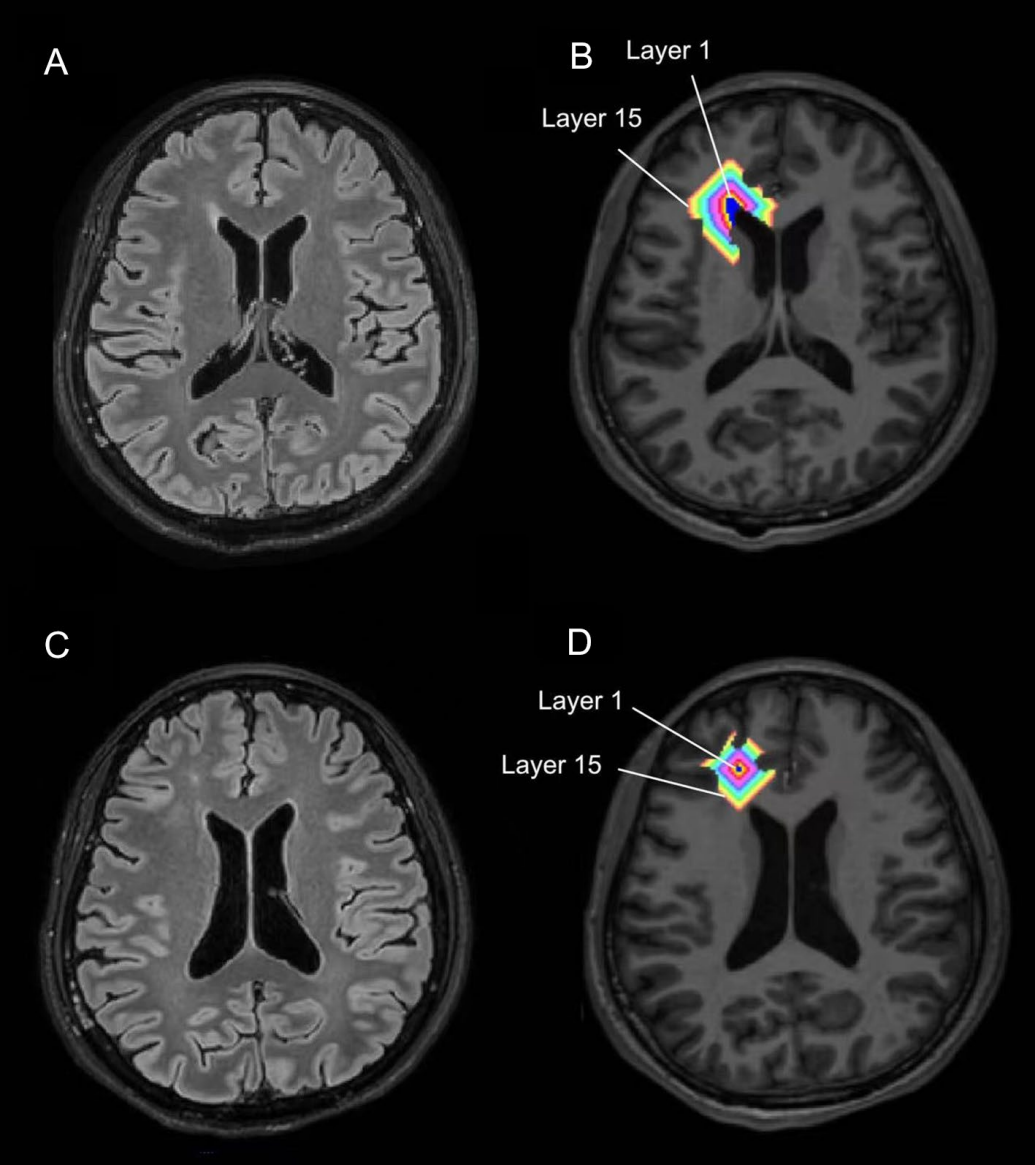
The spatial relationship between PVWMH, DWMH, and their corresponding NAWM layer masks. The dark blue represents WM lesions. The innermost yellow layer surrounding the WMH represents layer 1, and the outermost red layer represents layer 15. Each color represents a layer. A Periventricular white matter hyperintensity (PVWMH) on T_2_-FLAIR weight image (WI); B WMH and NAWM layer masks for PVWMH; C deep white matter hyperintensity (DWMH) on T_2_-FLAIR WI; D WMH and NAWM layer masks for DWMH
